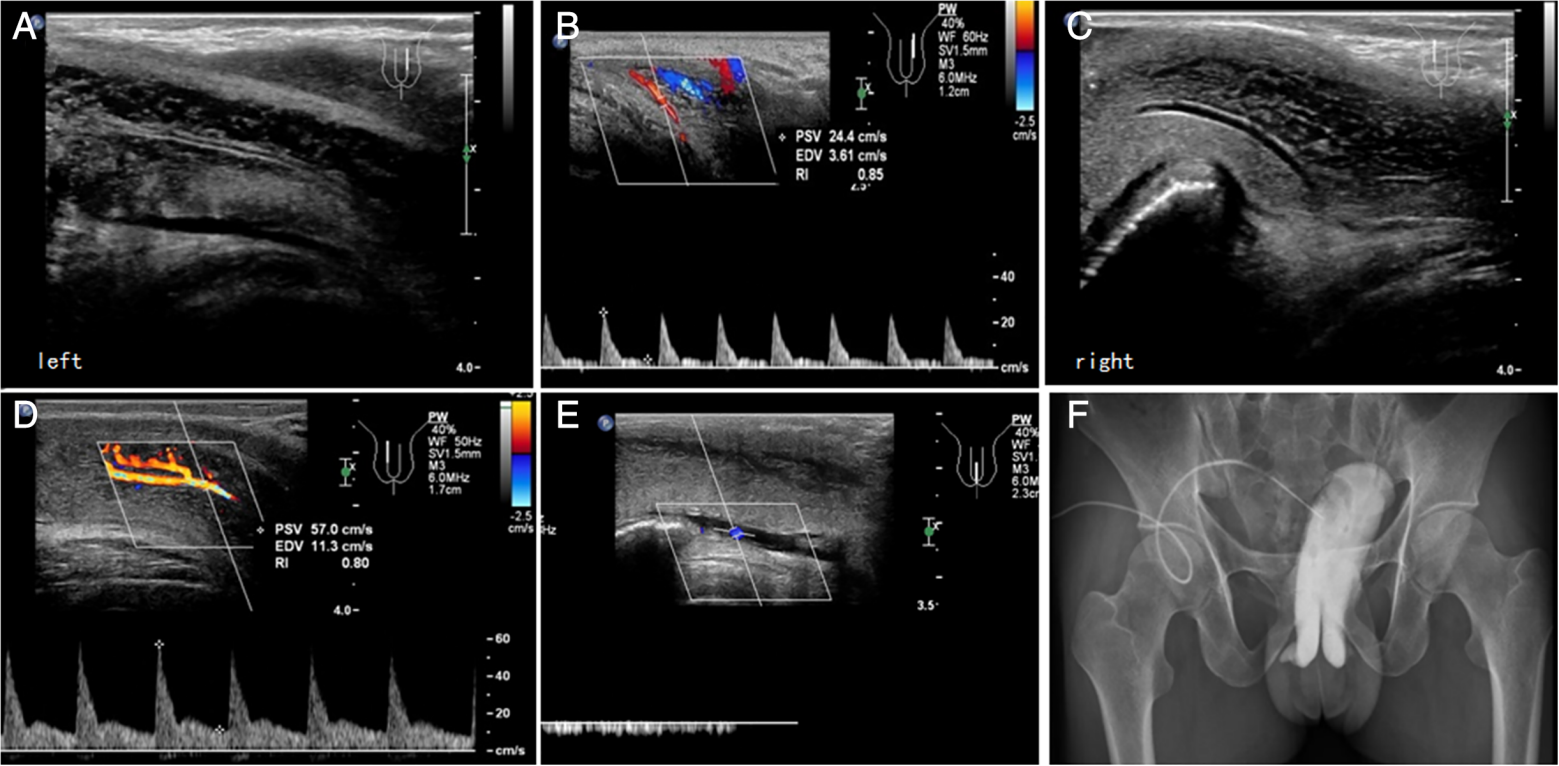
No cavernosal leakage in another 26‐year‐old man who had a false‐positive diagnosis of PPDS. A and B, The spectrum of the left cavernous artery that had a finer lumen suggested PSV < 30 cm/s, EDV < 5 cm/s, and RI = 0.85. C and D, The spectrum of the right cavernous artery that had a wider lumen suggested PSV > 30 cm/s, EDV > 5 cm/s, and RI = 0.80. E, The spectrum of the deep dorsal vein revealed a low‐velocity discontinuous blood flow. F, The cavernosography showed no obvious enhancement of the penile venous drainage. EDV indicates end‐diastolic velocity; PPDS, pharmaco‐penile duplex sonography; PSV, peak systolic velocity; RI, resistance index.
